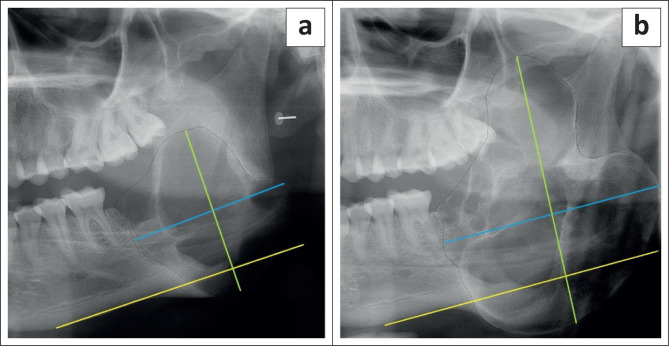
Size measurement of an ameloblastoma on panoramic radiograph at initial presentation (a) and after 17 months (b). The yellow line represents the inferior border of the mandible. The blue line represents the anterior-posterior dimension and the green line the superior-inferior dimension.
